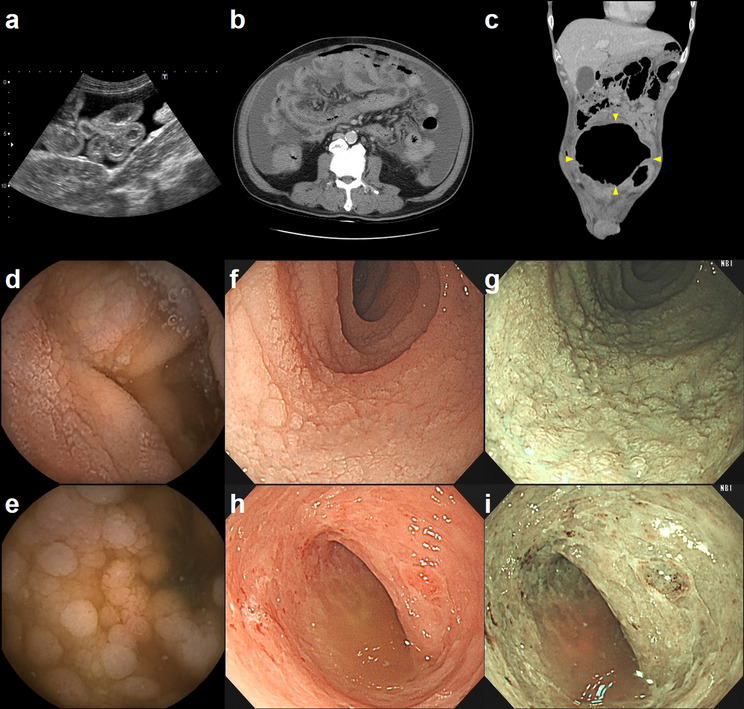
Representative images of MEITL. a Abdominal ultrasound image; b, c Abdominal computed tomography images, arrowheads indicate the location of the cavity; d, e Capsule endoscopy images; f-i Single-balloon enteroscopy images: f, g mosaic mucosal pattern, white light and narrow band imaging; h, i diffuse erosions with scattered shallow ulcers, white light and narrow-band imaging
